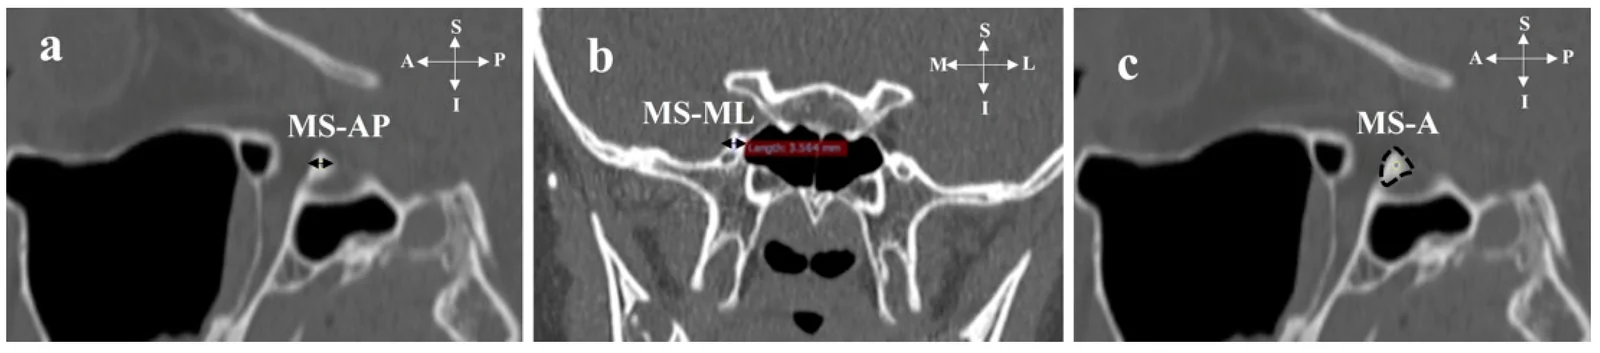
Computed tomography-based measurement of maxillary strut morphometric parameters. (a) MS-AP, the maximum anteroposterior dimension measured on a sagittal reformatted image; (b) MS-ML, the maximum mediolateral thickness measured on a coronal reformatted image (Length: 3.564 mm); and (c) MS-A, the cross-sectional area delineated on an oblique sagittal image perpendicular to the long axis of the strut. MS, maxillary strut; MS-AP, maxillary strut anteroposterior diameter; MS-ML, maxillary strut mediolateral diameter; MS-A, maxillary strut cross-sectional area; A, anterior; P, posterior; S, superior; I, inferior; M, medial; L, lateral.
